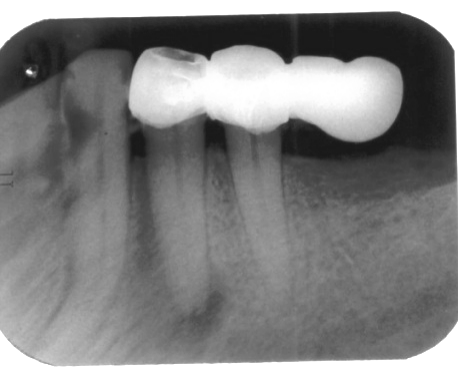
Tooth 34 with periapical contoured radiolucency
